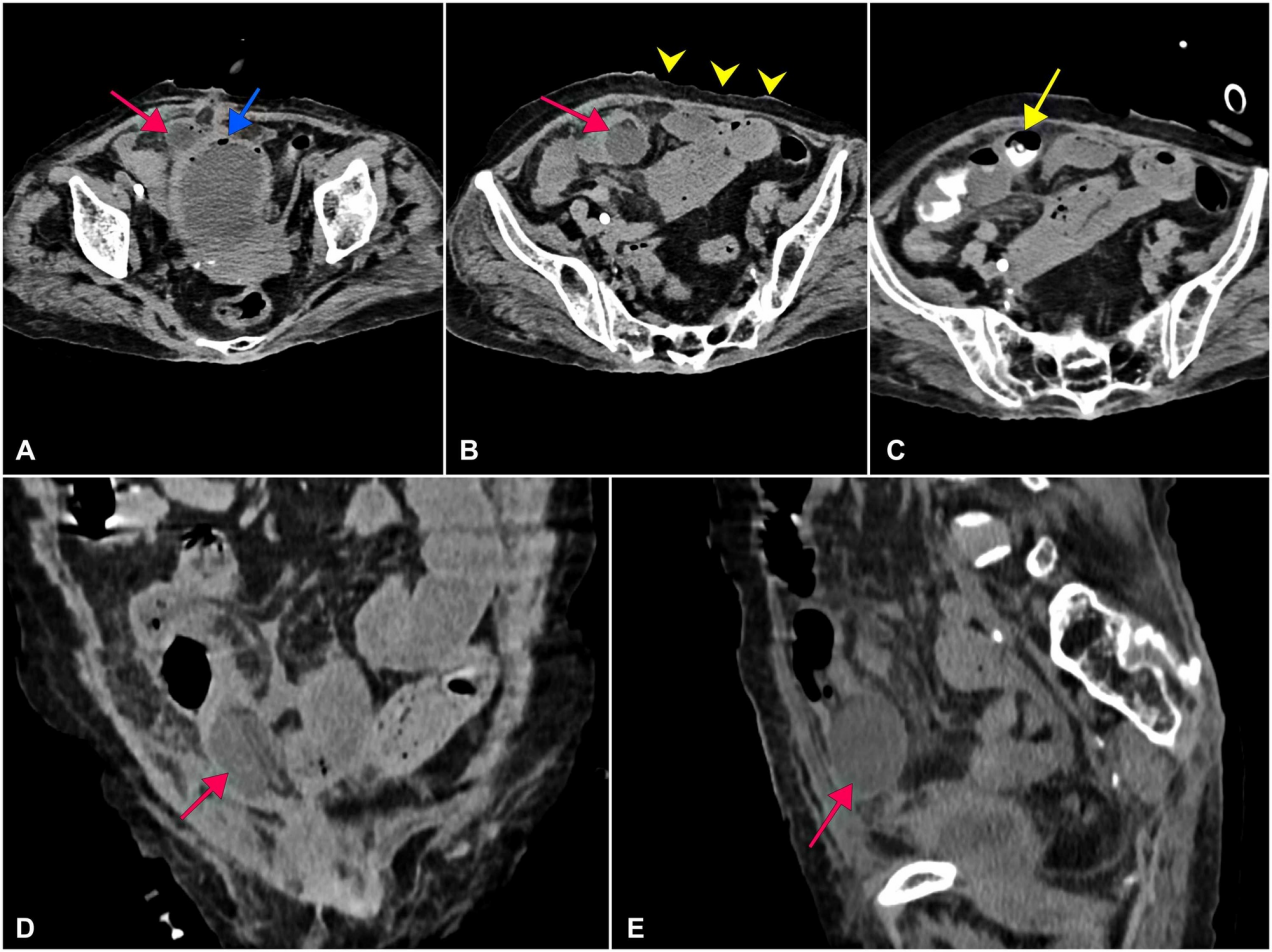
CT images showing the Foley bulb in the ileal loop (red arrows). The blue arrow shows the distended bladder with air specks. The yellow arrowheads show the radiation changes in the skin and adhered bowel loops just underneath the abdominal wall. The yellow arrow shows contrast flowing from the tip of the Foley catheter into the ileal loops. (A-C) Axial sections. (D) Coronal section. (E) Sagittal section.
